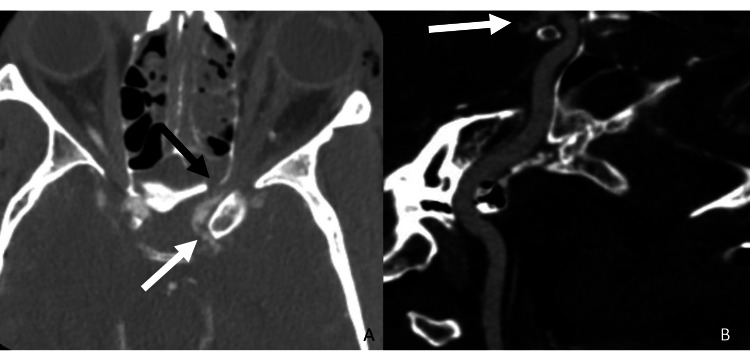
Supraclinoid ICA traumatic vessel injury Axial CTA neck (A) and curve reformat (B) demonstrate vessel irregularity and stenosis along the left supraclinoid ICA (white arrow) adjacent to the sphenoid sinus wall fracture (black arrow). ICA: internal carotid artery; CTA: computed tomography angiography
